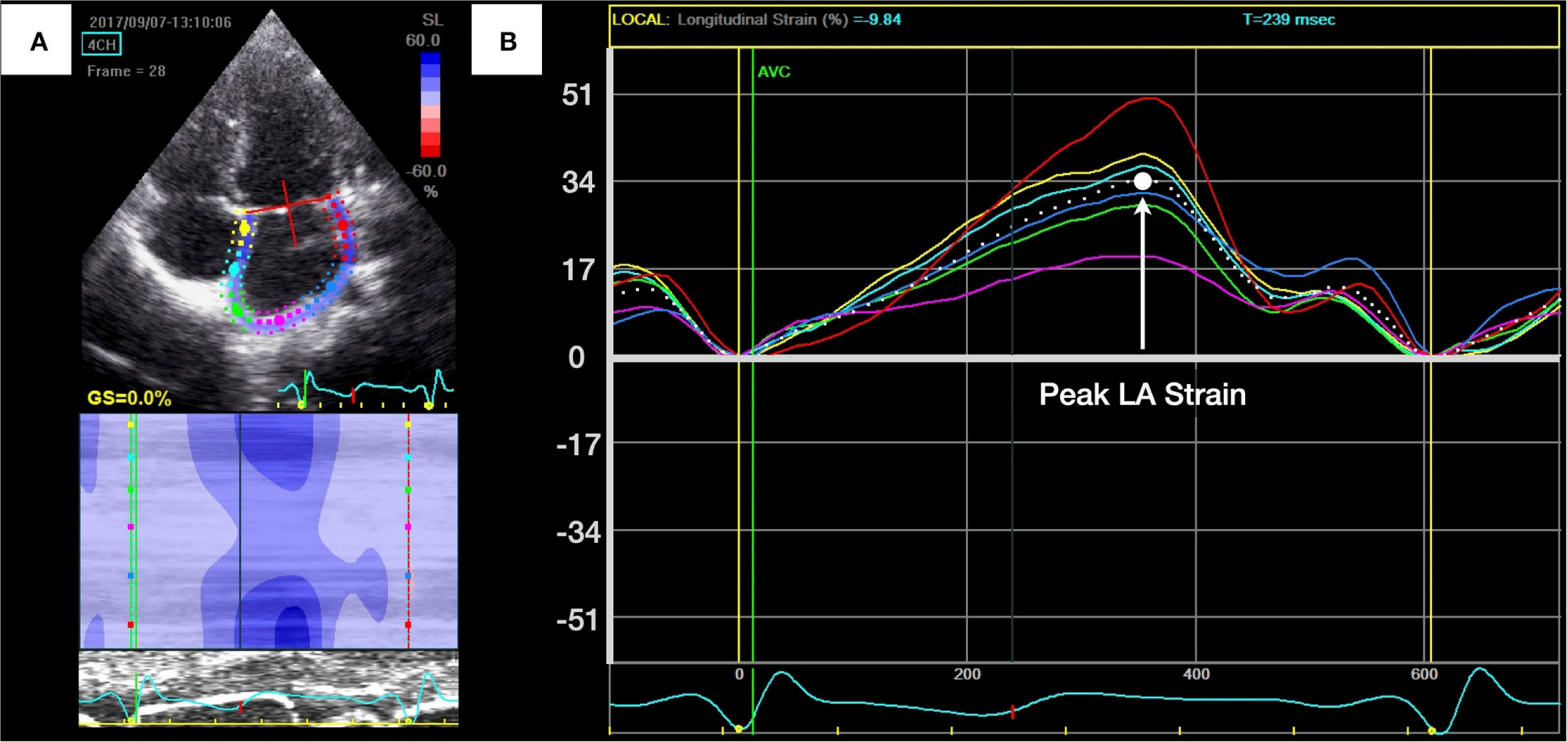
Measurement of left atrial (LA) longitudinal peak systolic strain. (A) Apical four-chamber view of the left atrium with the region of interest. (B) The peak LA longitudinal strain during reservoir phase was identified as the highest point and was calculated by averaging measurements of six curves.
